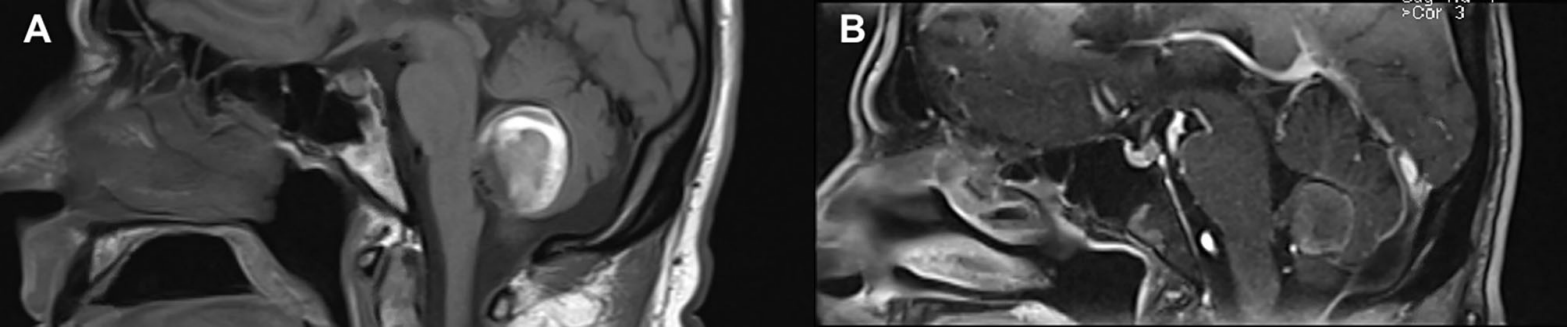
MRI comparison before treatment (A) and at 9 months follow up (B). In follow up, there is no further filling of the giant aneurysm, and the thrombosed aneurysm sac measures 2.1 cm × 2.3 cm × 2.2 cm compared to 3.1 cm × 3.1 cm × 2.8 cm prior to treatment.
